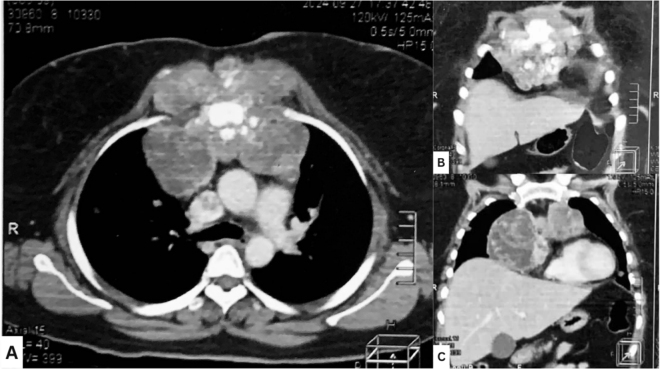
CT scan pre-chemotherapy. (A) Transverse cut. (B, C) Coronal cut demonstrating the extension of the mass, involving the presternal, parasternal, and retrosternal regions of the chest wall.
